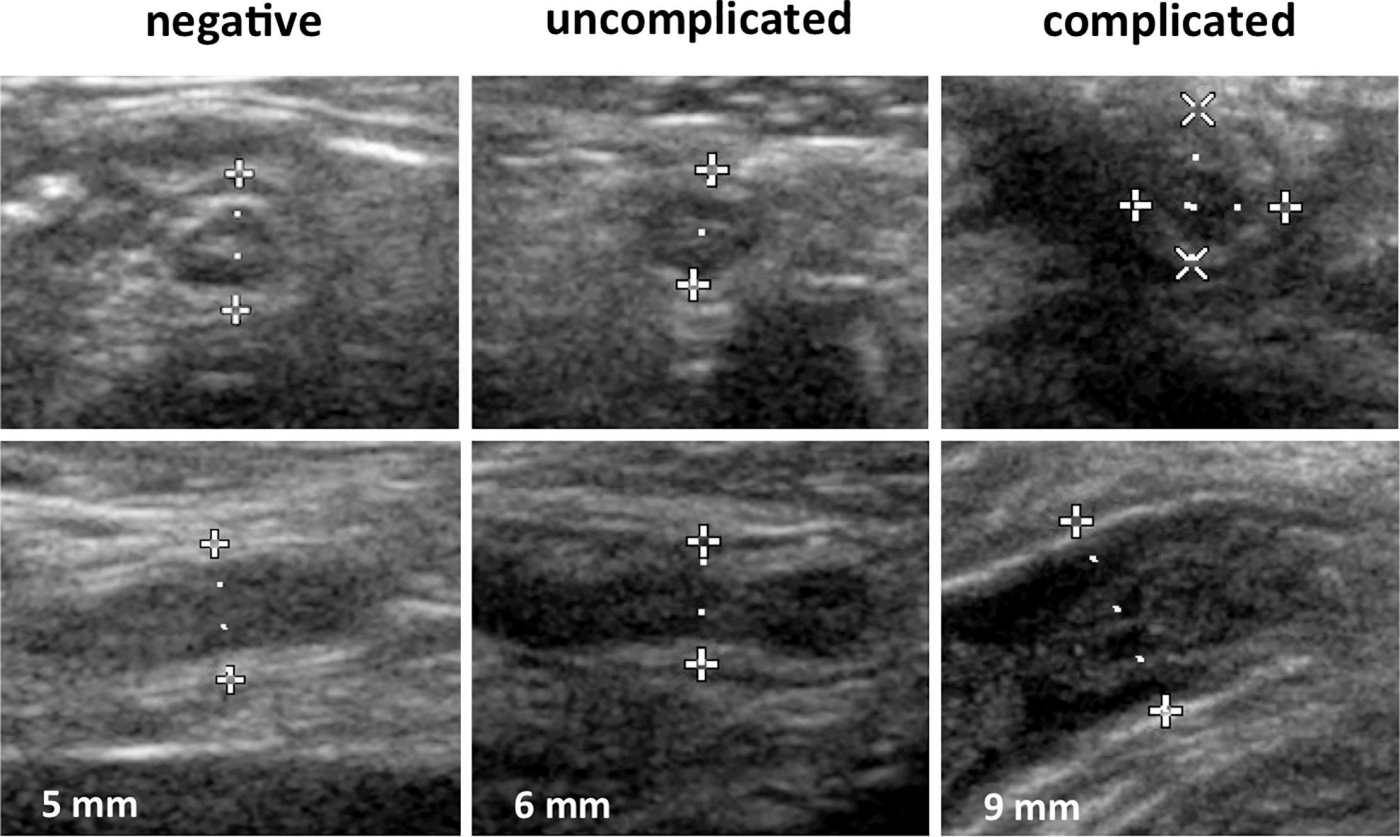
Sonographic images of appendices from 8 years old female patients without inflammation, with uncomplicated and with complicated appendicitis; cross and longitudinal sections, respective maximum diameters [mm].
